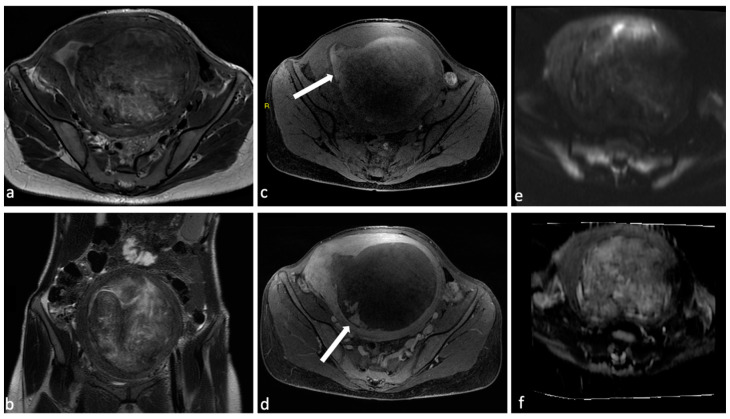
Leiomyoma with red degeneration. A 43-year-old woman with known submucosal myoma with recent onset of AUB and pelvic pain. On MRI, T2 axial (a) and coronal (b) sequences show enlargement of the known submucous leiomyoma with inhomogeneous signal intensity and peripheral rim hypointensity. The leiomyoma is characterized by signal hyperintensity on T1WI (c, arrow) and a small enhancing solid portion on post-contrast T1WI (d, arrow). There is no significant restricted diffusion/hypointensity on the ADC map (e,f).
